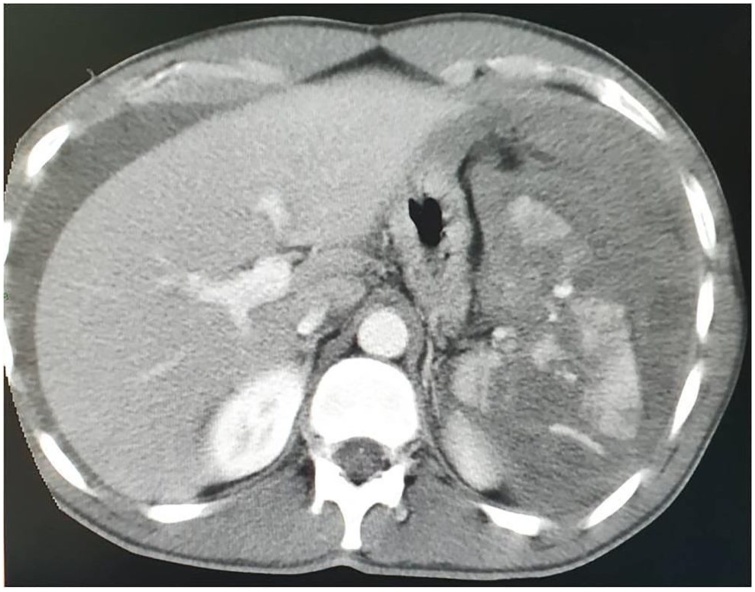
Abdominal CT scan showing splenic rupture with hemoperitoneum.
